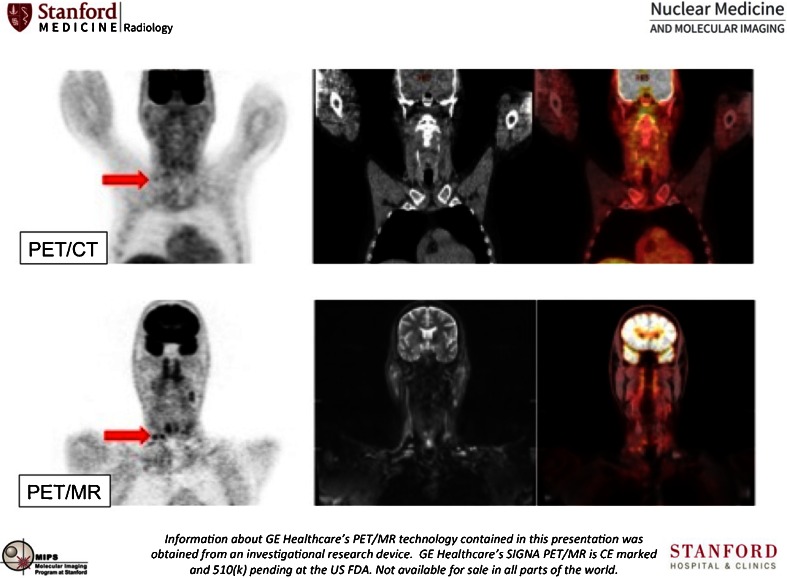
Comparison of TOF PET/CT and TOF PET/MRI for the detection of cervical lymph nodes [3]
